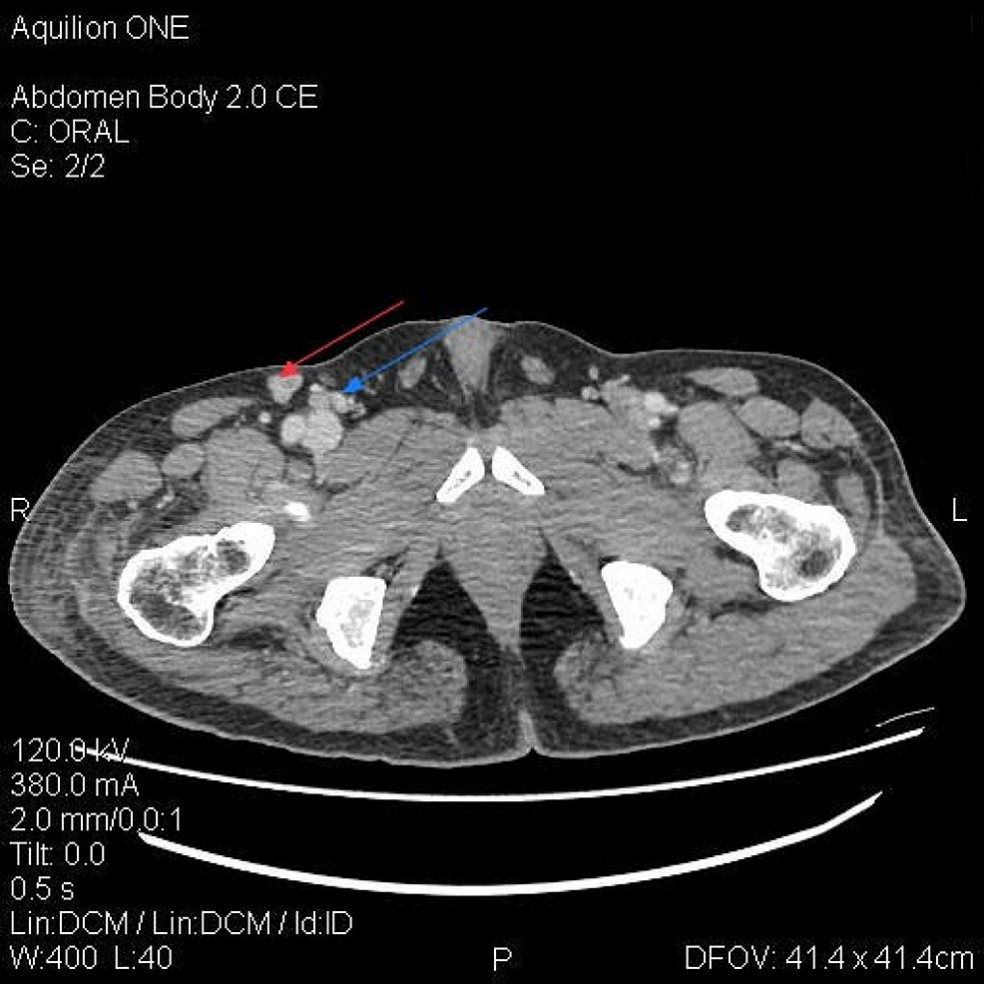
CT pelvis with contrast showing enlarged right inguinal (red arrow) and right external iliac lymph nodes (blue arrow) with mild subcutaneous strandy changes in the right upper thigh.
